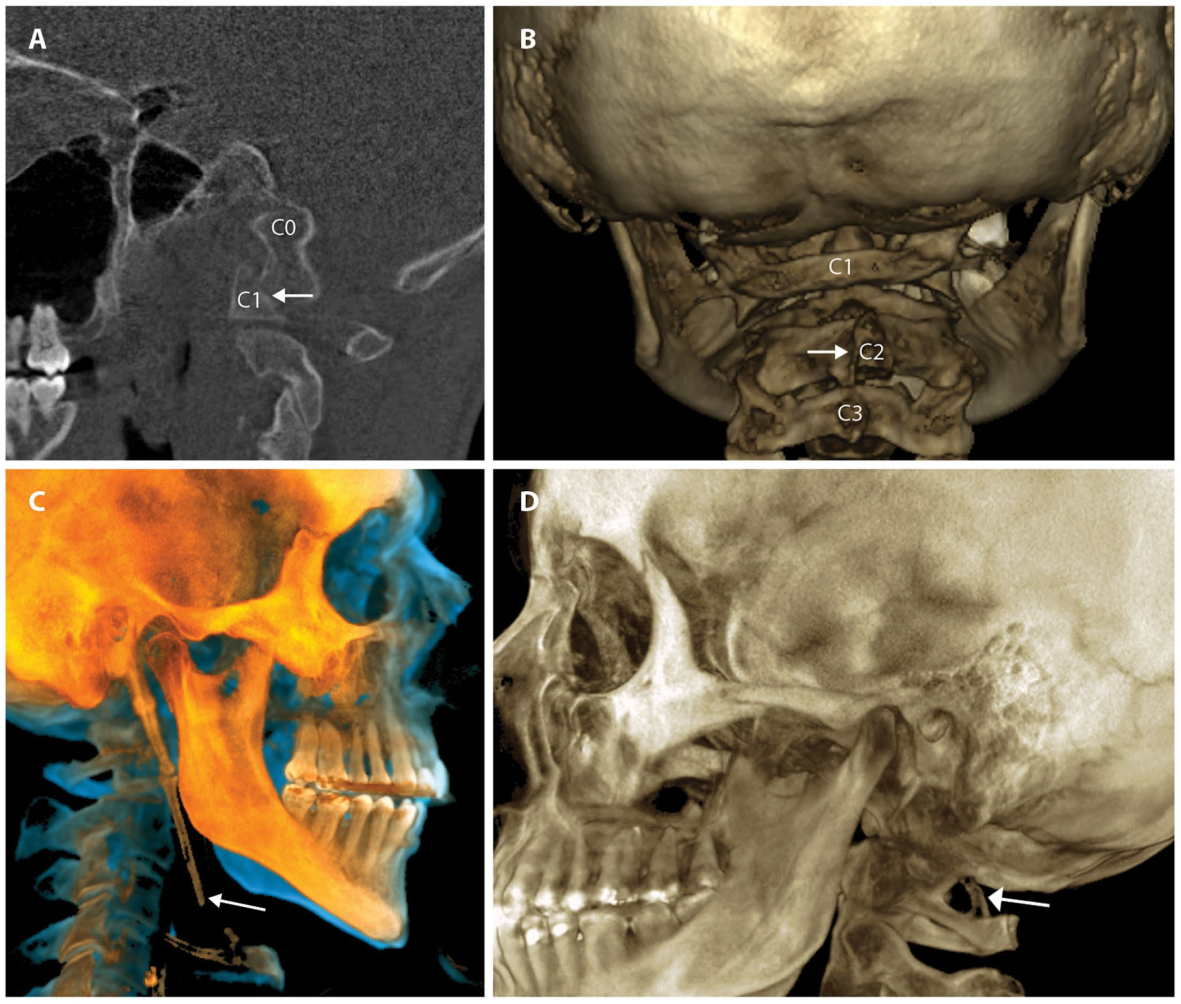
Common pathology seen by upright cone beam CT scan of head and neck. (A) CO-C1 instability. (B) Cervical spine misalignments. (C) Elongated styloid. (D) Ponticulus posticus.
